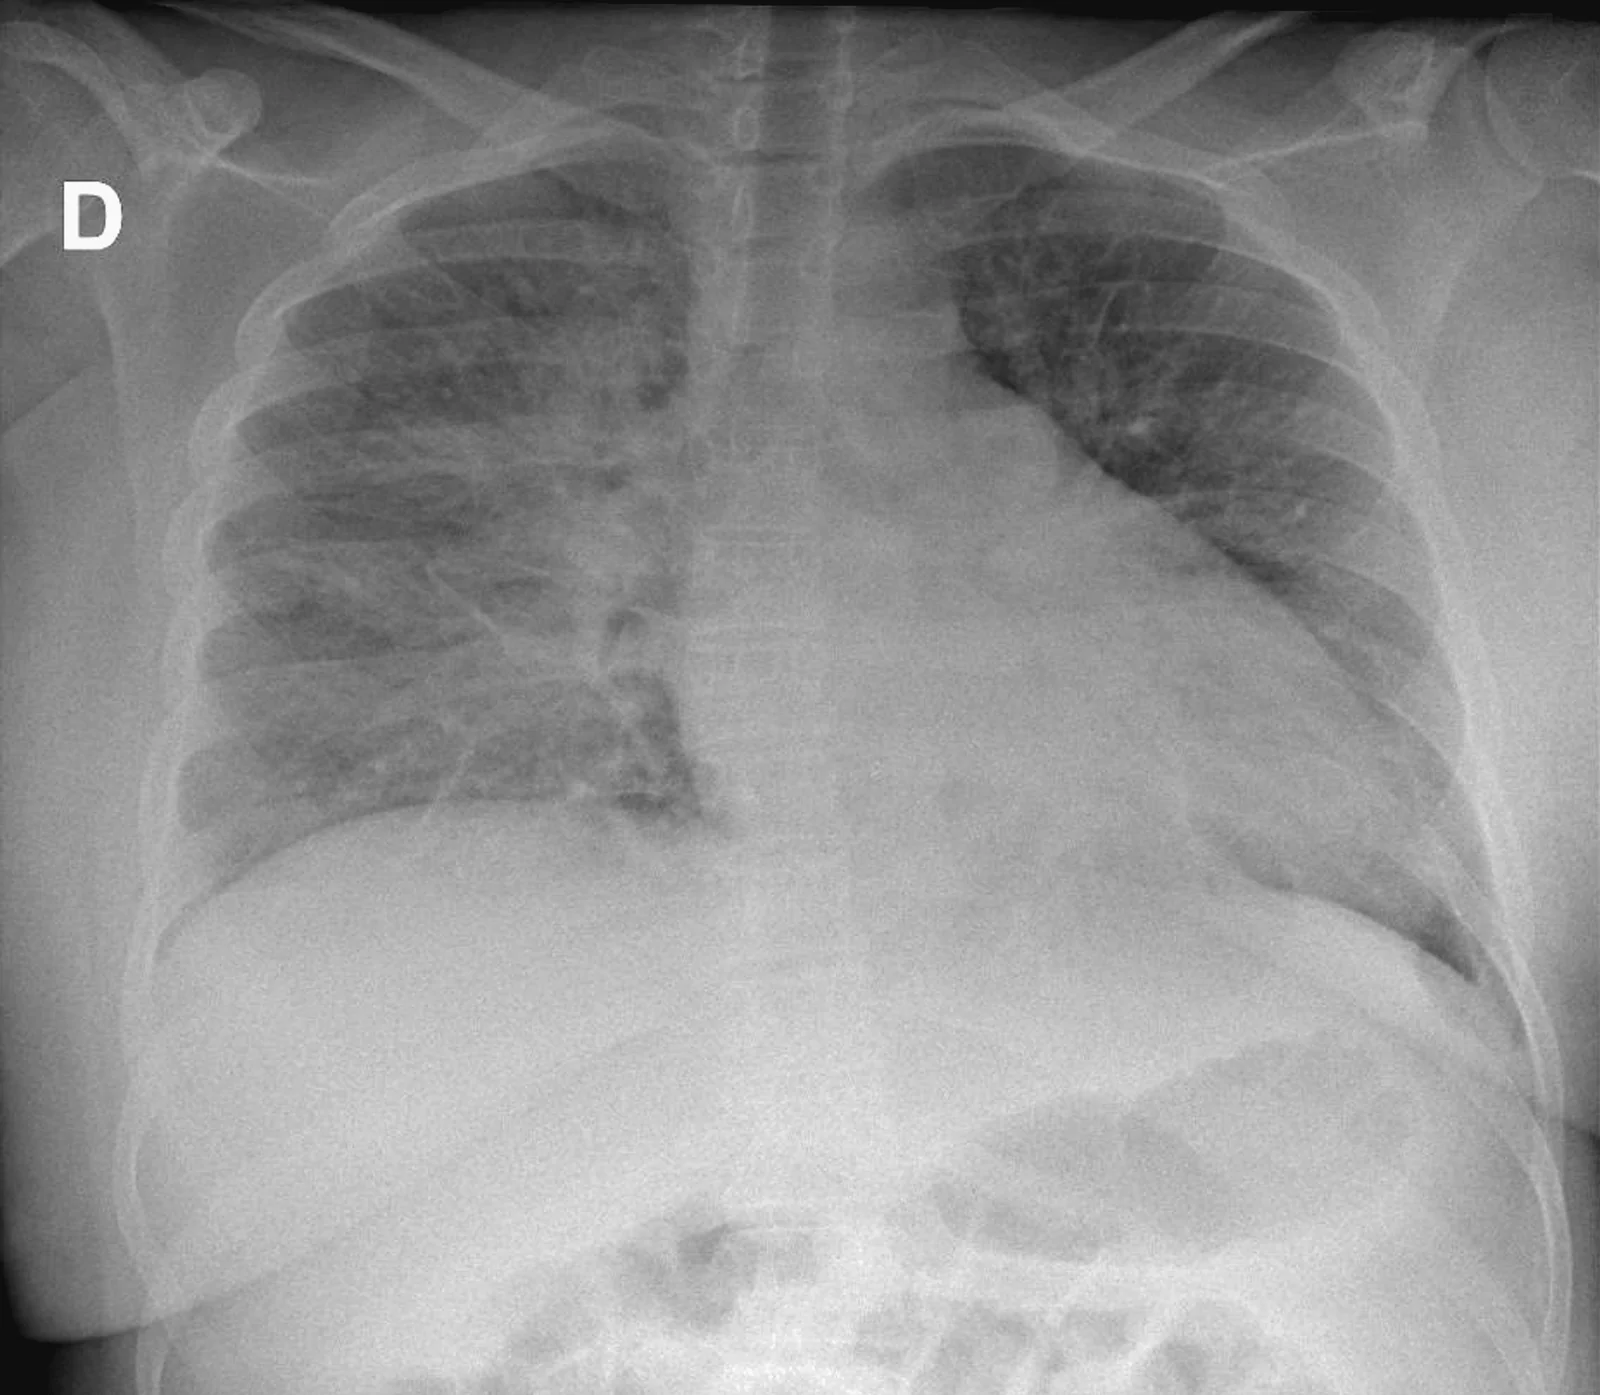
Chest X-ray Chest radiograph obtained on Day 2, showing diffuse bilateral alveolar-interstitial infiltrates predominantly involving the perihilar and lower lung fields, consistent with non-cardiogenic pulmonary edema.
